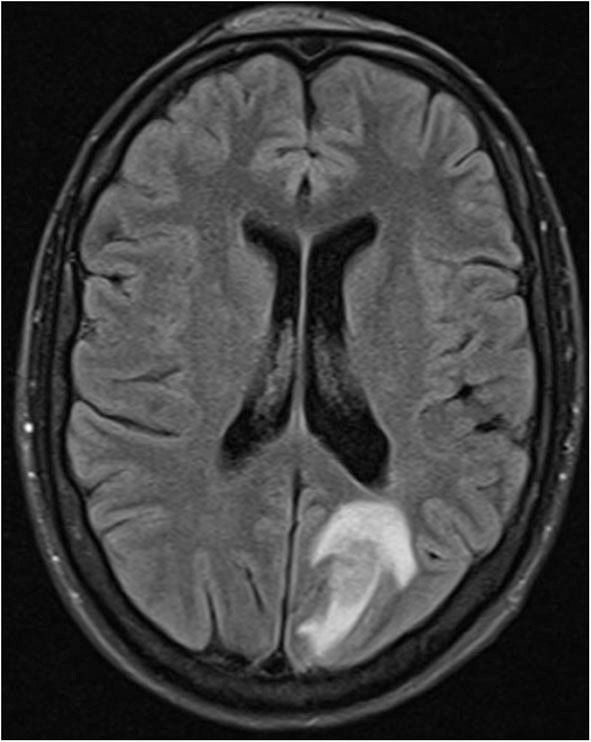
Hyperintense lesion with perifocal edema left occipital in fluid-suppressed T2 MRI technique (Fluid-attenuated inversion recovery (FLAIR) sequence).
